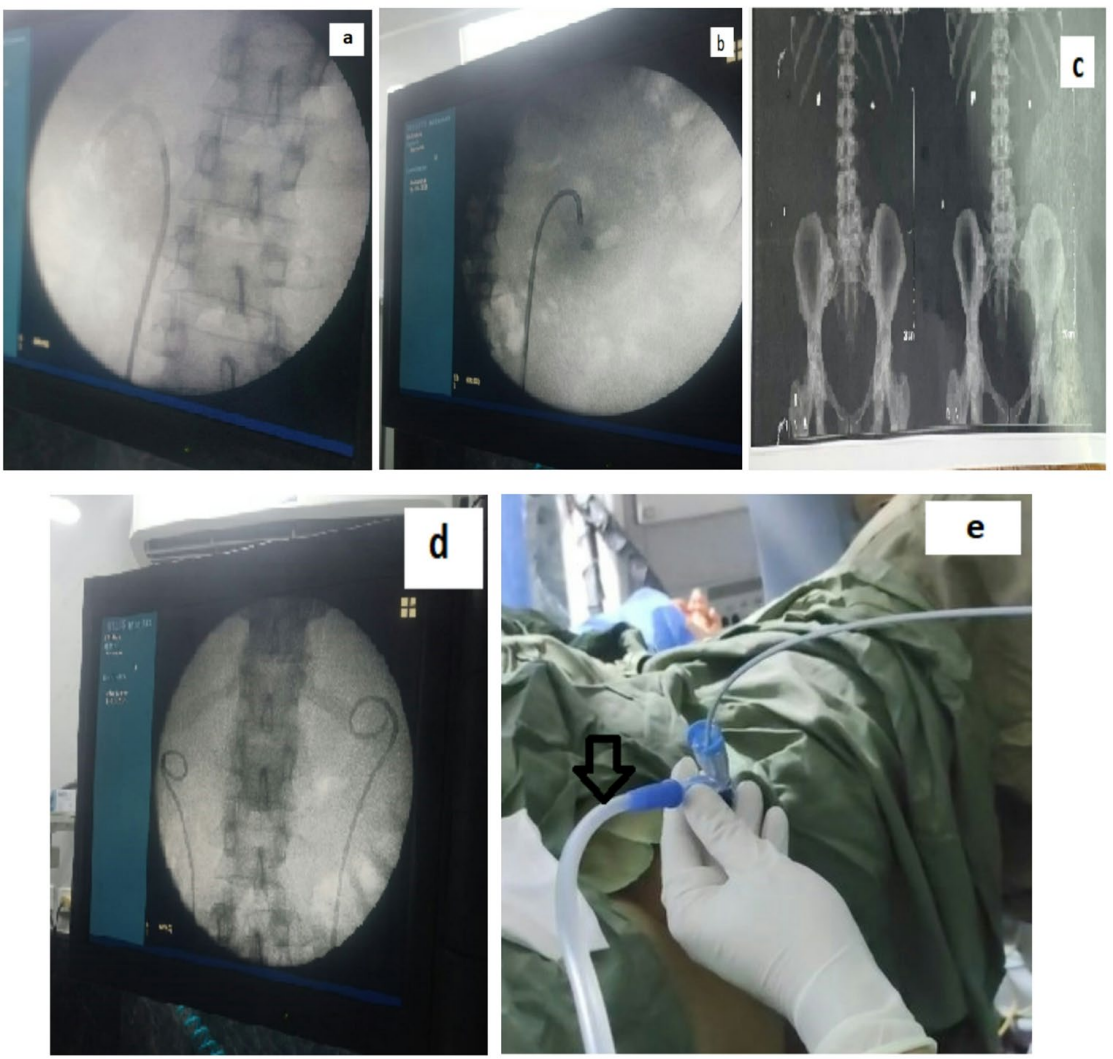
(a): flexible URS going through bended tip of Novel ureteral access sheath (b): bended tip of novel access sheath in close proximity to the stone. (c) CT” KUB " showing bilateral renal stone (d) Fluoroscopic image of a stone free case with bilateral jj after flexible URS using novel access sheath. (e) Suction port of novel access sheath connected to vacuum suction device
